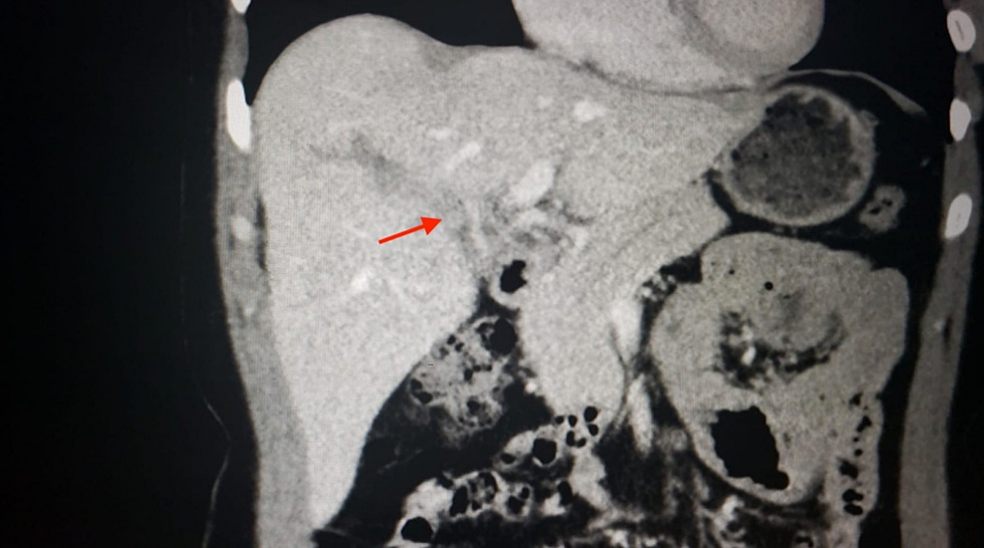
CT imaging of PVT in a COVID-19 positive patient. CT, computed tomography; PVT, portal vein thrombosis; COVID-19, coronavirus disease 2019
